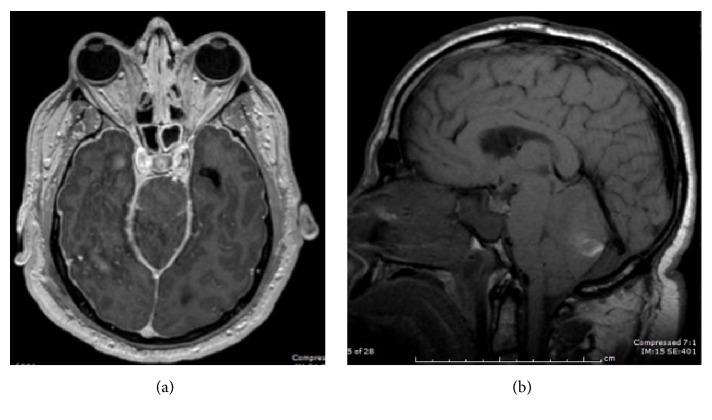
MRI brain was performed on the same date of the CT head. It confirmed cerebellar haemorrhage and mass effect on the 4th ventricle and brainstem. (a) Axial T1 SPGR postgadolinium MR image shows diffuse dural enhancement. (b) Sagittal T1 MR image of the brain shows “sagging” midbrain, flattening of the pons against the clivus, and mild cerebellar tonsillar descent. The findings in (a) and (b) are consistent with intracranial hypotension.
